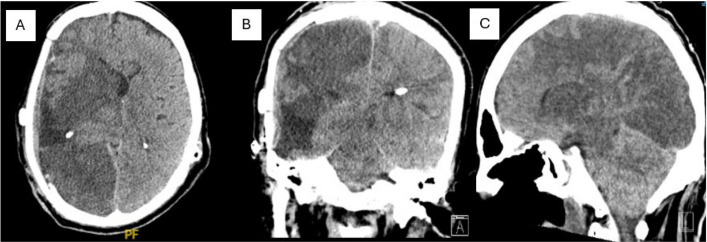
MRI of Malignant Cerebral Edema. A. Axial CT B. Coronal CT C. Sagittal CT
